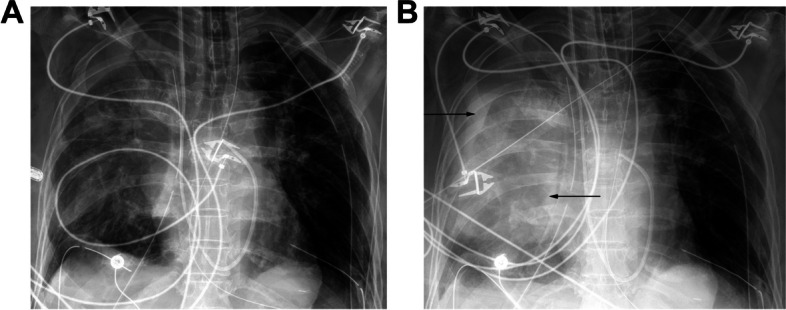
60-year-old female who developed hemothorax immediately after single lung transplant. A Frontal chest radiograph obtained immediately after surgery shows right sided lung transplant with support devices, a layering pleural effusion overlying the lung apex, and hazy airspace opacities. B Frontal chest radiograph obtained later the same day shows rapid opacification of the right lung transplant with contralateral mediastinal shift due to a large layering effusion (arrows). Findings were suspicious for rapidly developing hemothorax. The patient was taken to surgery, confirming hematoma in the pleural space, with subsequent evacuation and resolution (not shown)
